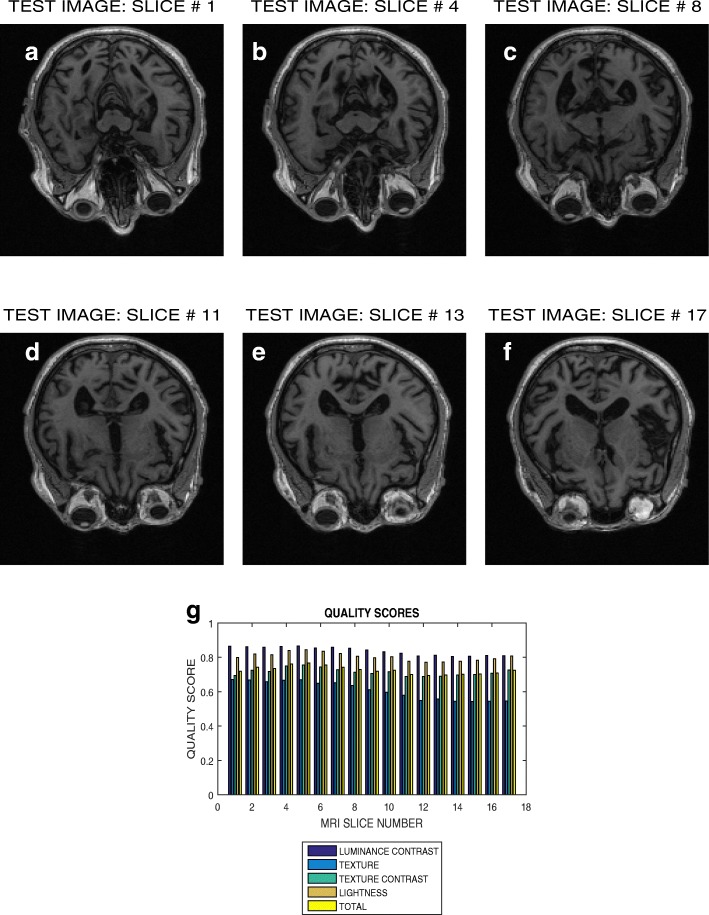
Six slices with indices (a) 1, (b) 4, (c) 8, (d) 11, (e) 13 and (f) 17 in a T1 MPRAGE pulse sequence MRI volume data from ADNI, (g) luminance contrast, texture, texture contrast, lightness and total quality scores of 17 successive slices in the MRI volume data
